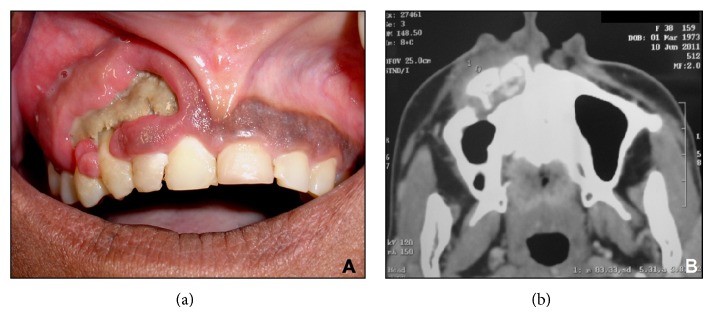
Clinical (a) and radiographic (b) image upon presentation. (a) Note the exposed bony sequestrum and significant destruction of gingiva and alveolar mucosa in the maxillary right quadrant. (b) Axial view (CT scan); note the alveolar bone destruction on the maxillary right quadrant, the surrounding radiolucent halo, and the partial destruction of the front wall of the right maxillary sinus.
